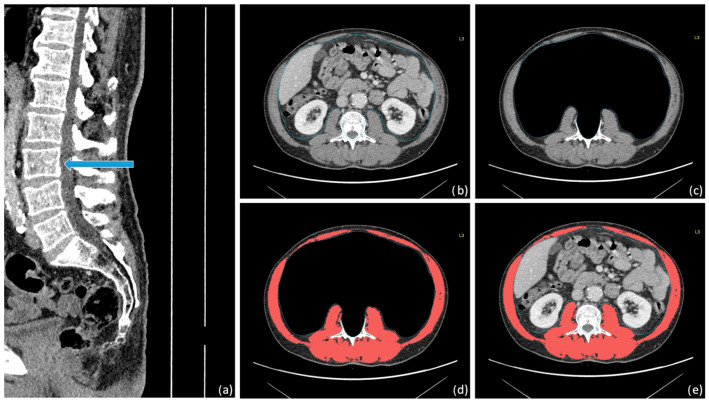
(a) CT scan at the L3 vertebra level (blue arrow); (b) segmentation of visceral fat and organs; (c) removal of visceral fat and organs; (d) semi-automatic muscle segmentation of the SMI at the L3 level (red); (e) summary view of all regions of interest.
